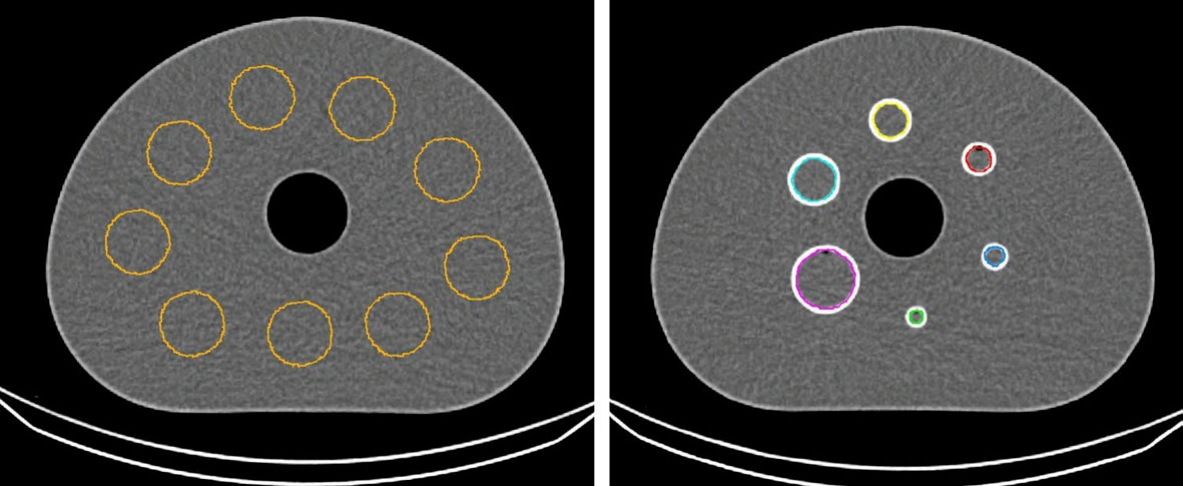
Delineation of the background VOI (left) and the spheres (right) on the corresponding CT image
